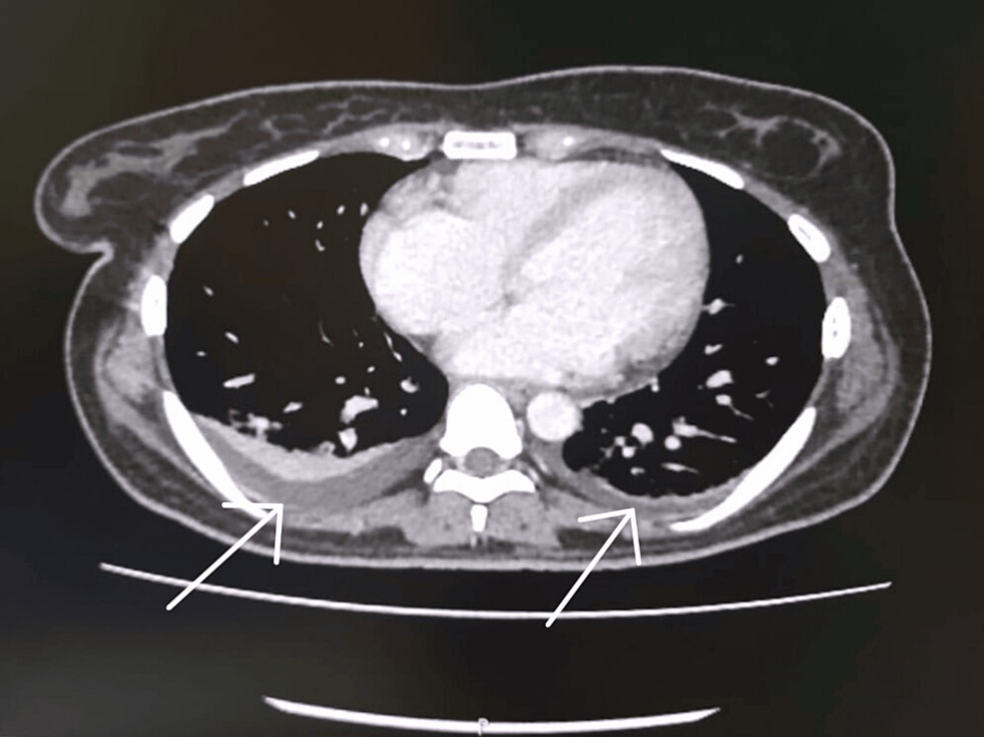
Contrast-enhanced CT of the chest showing bilateral pleural effusion with adjacent collapse consolidation (white arrows) No lung masses, nodules, or cavitatory lesions. No hilar or mediastinal lymphadenopathy CT: computed tomography
